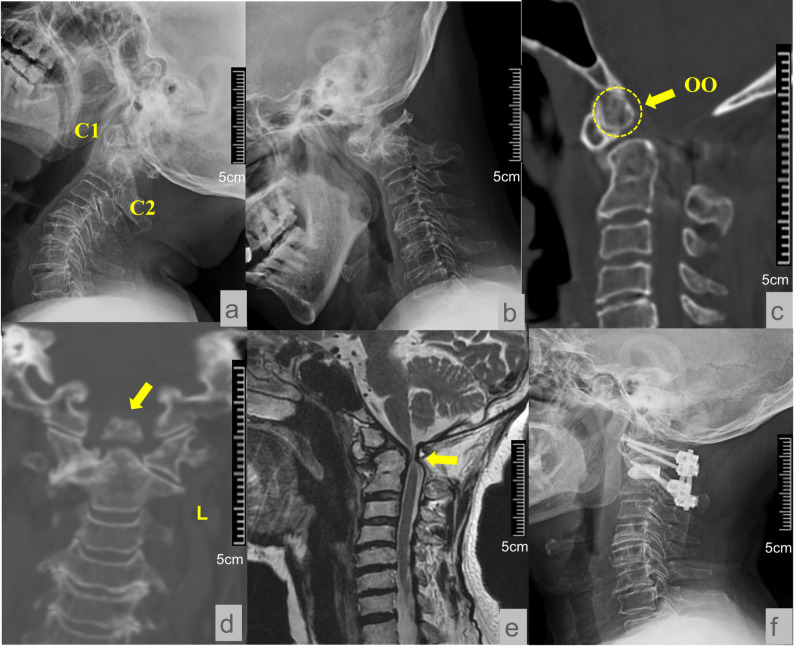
A 76-y/o female OO patient. a&b. preoperative dynamic X-ray showed OO deformity and atlantoaxial dislocation; c. preoperative CT with sagittal images showed OO deformity(within the dashed circle): the apex of the odontoid process is separated from the base of the C2 vertebral body; d. preoperative CT with coronal images showed OO deformity(yellow arrow); e. preoperative MRI showed the severe compression of the spinal cord: the spinal cord is thinned(yellow arrow); f. postoperative X-ray showed that the atlantoaxial joint was reduced and fixed using screws, and a cage was placed between the atlantal and axial lateral masses for stabilization
